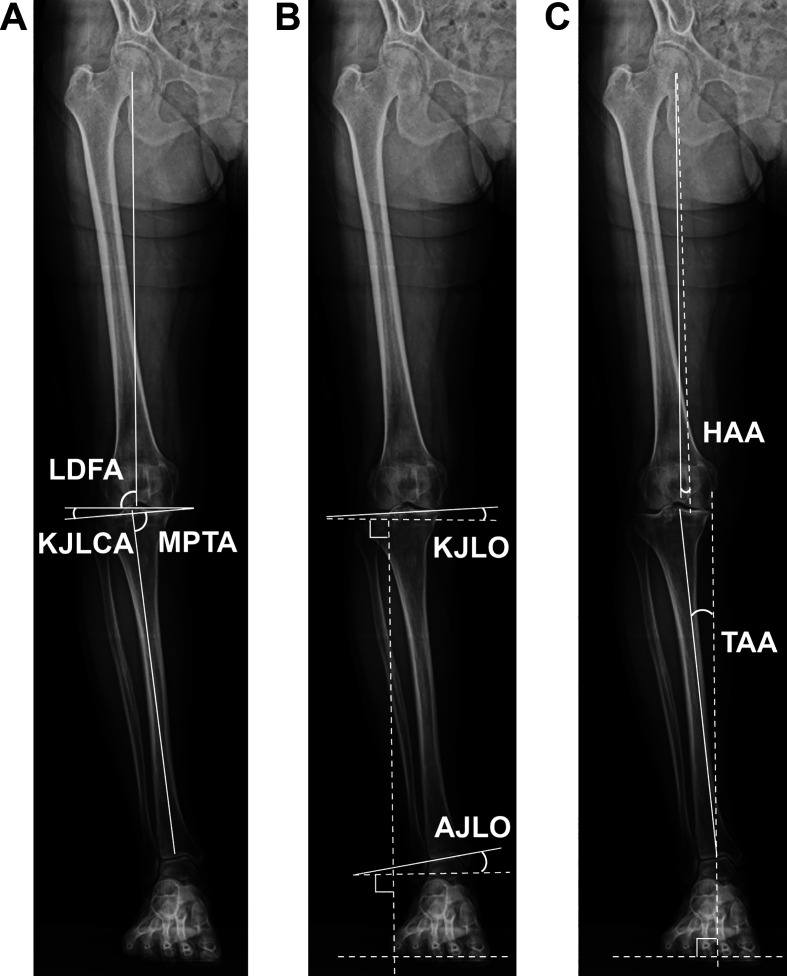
Radiographic measurements. (A) Lateral distal femoral angle (LDFA), medial proximal tibial angle (MPTA), and knee joint line convergency angle (KJLCA). (B) Knee joint line obliquity (KJLO) and ankle joint line obliquity (AJLO). (C) Hip abduction angle (HAA) and tibial axis angle (TAA).
